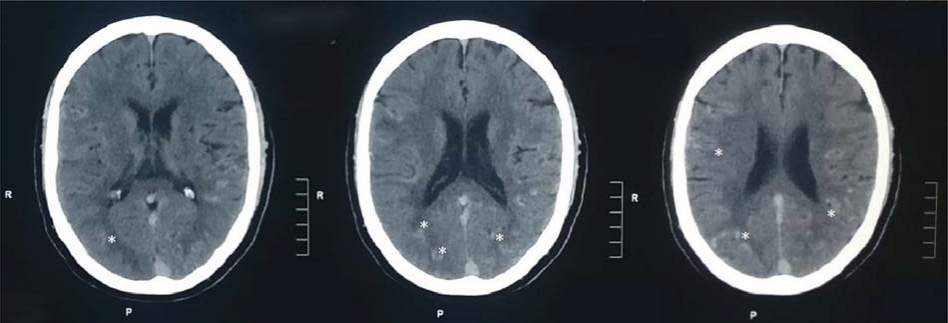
Plain CT head: plain CT head (axial sections) at the level of lateral ventricles show multiple linear areas of hyper-densities along cortical sulci of bilateral temporo-parieto-occipital lobes (shown by asterisks).
